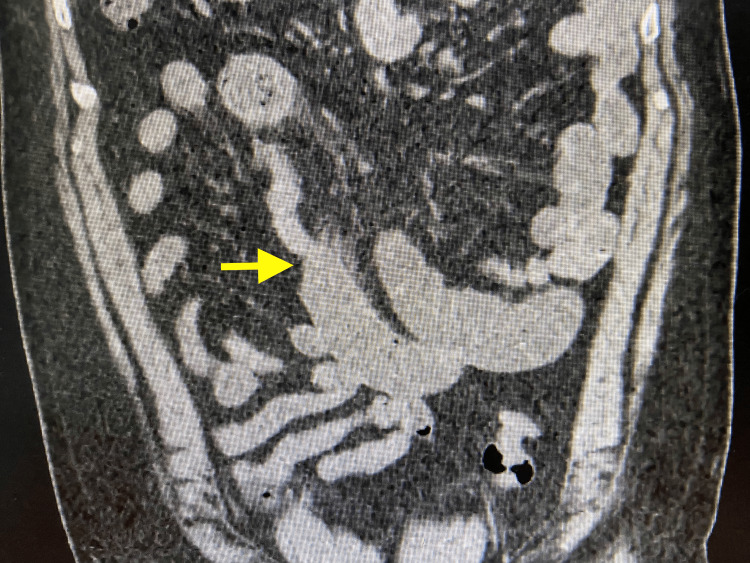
Transition point in the distal ileum on CT scan (arrow)
